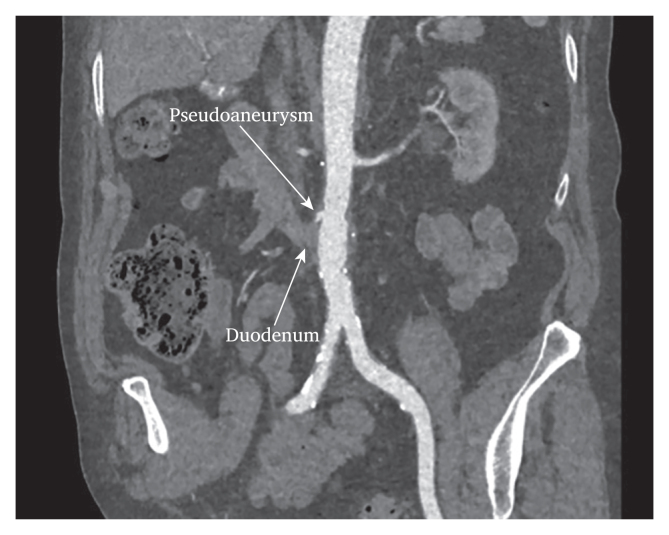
Multiple planar reconstruction of the computed tomography angiography scan two weeks pre-operatively for the secondary aorto-enteric fistula, showing a clear adhesion (arrow) between the duodenum and aorta.
